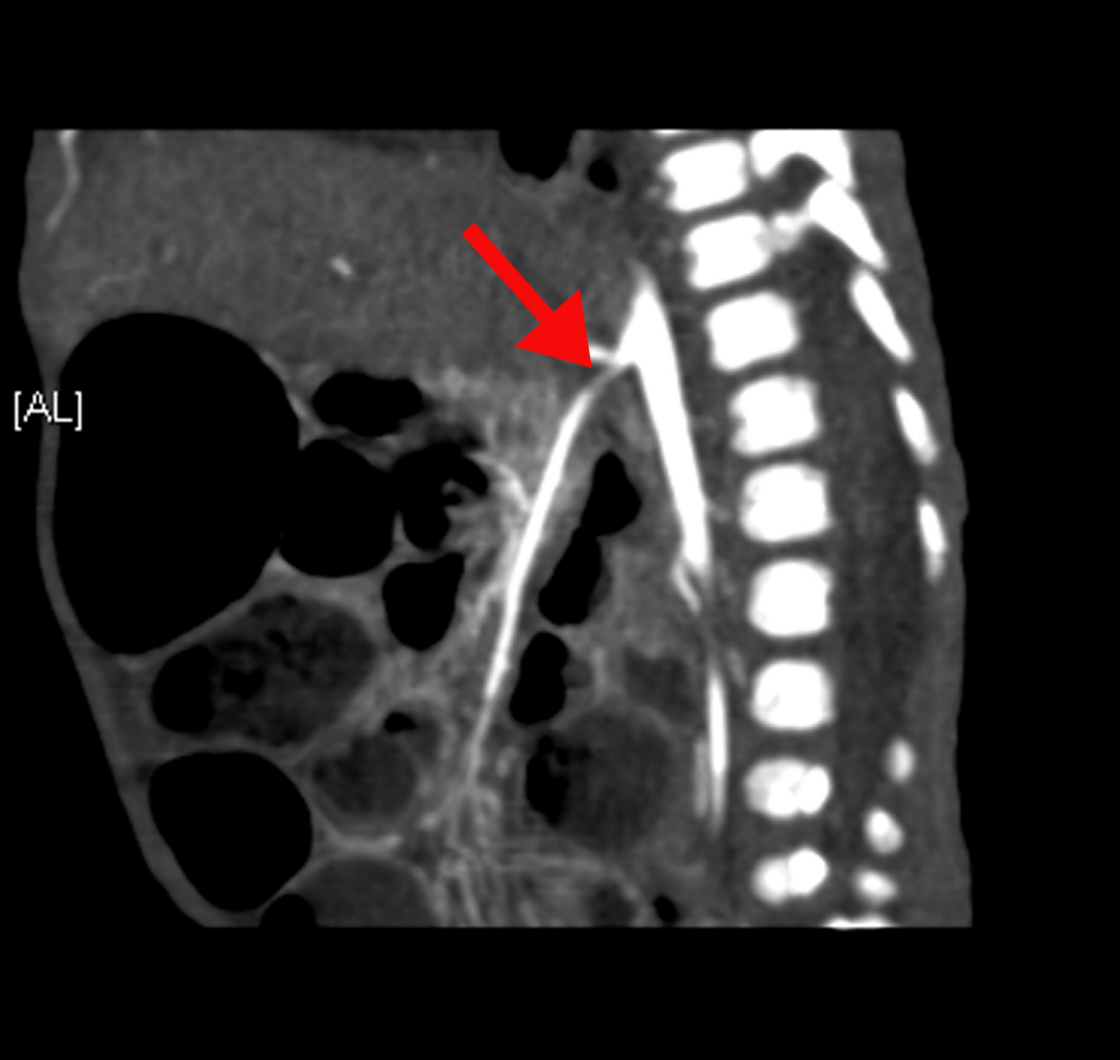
A computed tomography angiogram shows stenosis of the superior mesenteric artery (arrow).
